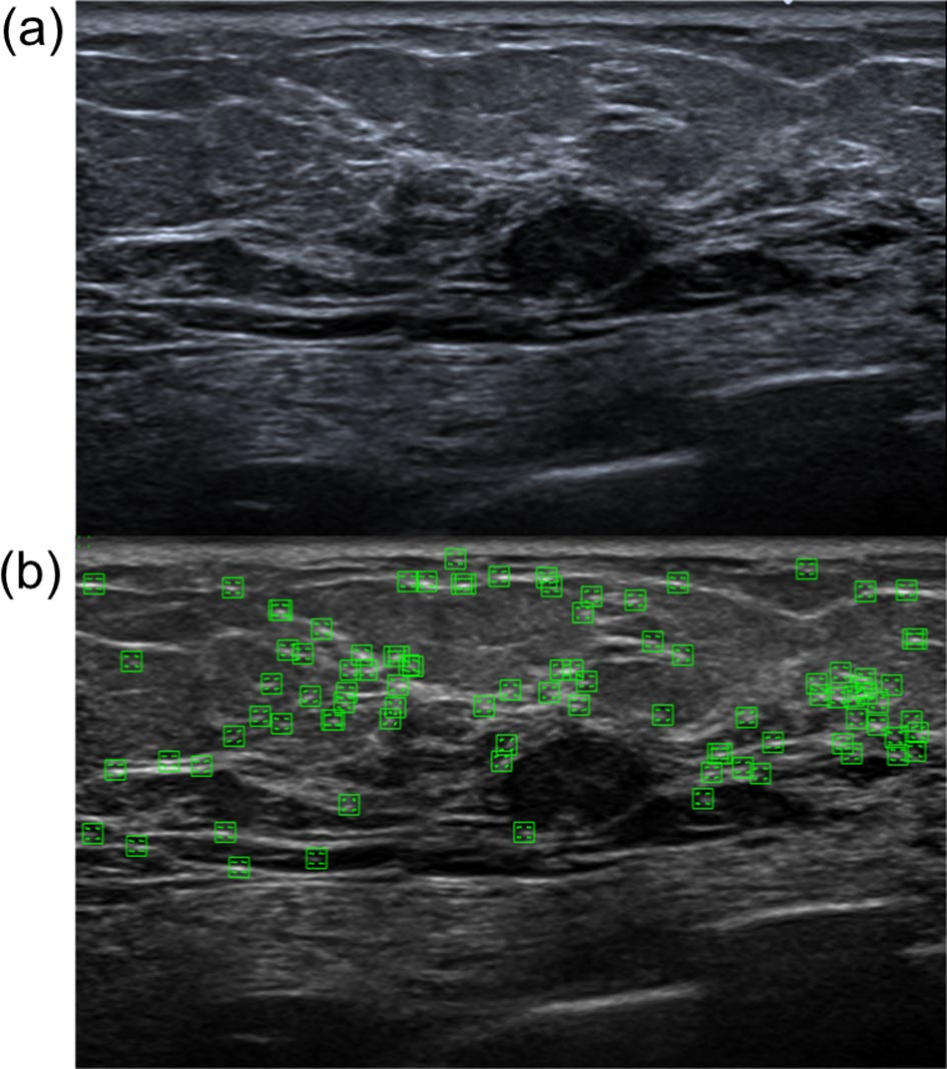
Visualised extracted feature vector from a benign US image by Histogram of Oriented Gradient (HOG). The probably benign (BI-RADS category 3) case: female, age 78 years, a left breast hypoechoic tumour was found and reported by ultrasound imaging, size: 11.7 × 6 mm, location: 2 o’clock from the nipple, distance: 2 cm from the nipple to the lesion. The fibroadenoma lesion was confirmed after a core needle biopsy, size: 1.2 × 0.1 × 0.1 cm. The extracted feature vectors through the HOG descriptor from the image are shown as corner points. (a) The US image of this case. (b) Green marks represent the positions of corner points obtained from the accelerated segment test (FAST).
